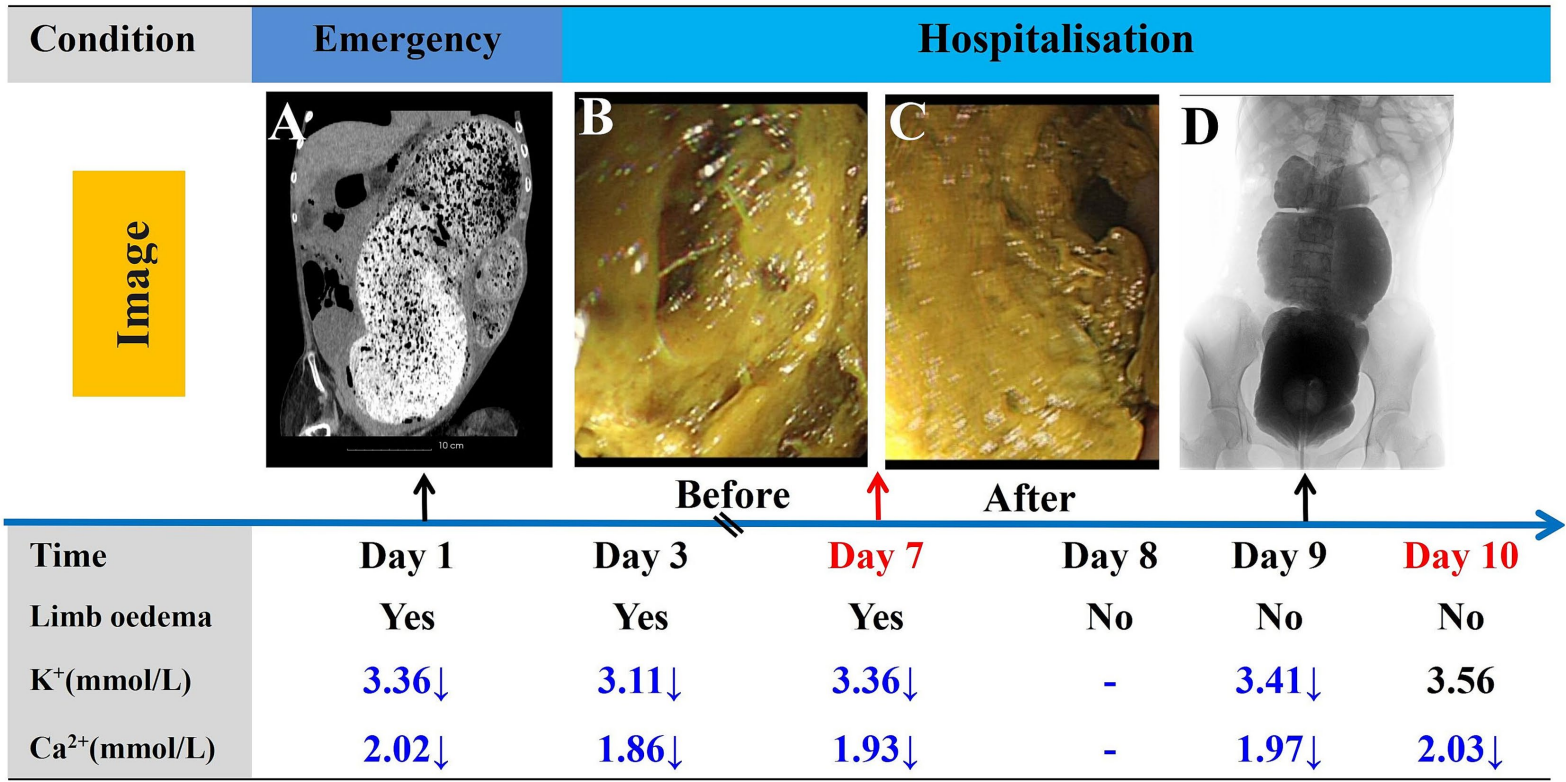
Timeline of the progression and key events during the diagnosis and treatment process. Abdominal CT images taken in the emergency department (A). Changes in intestinal fecal morphology before (B) and after (C) endoscopic treatment. Barium enema angiography following the resolution of intestinal obstruction (D). Post day 10, regular enemas administered by caretaker.
